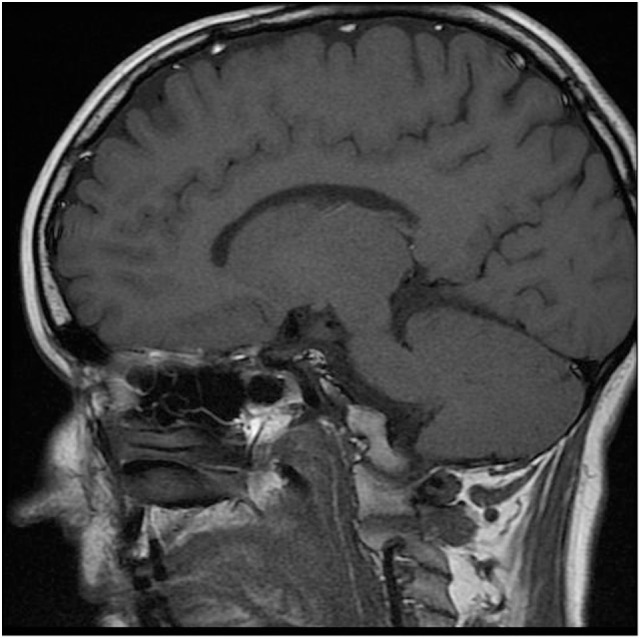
Magnetic resonance imaging of smaller pituitary gland.
